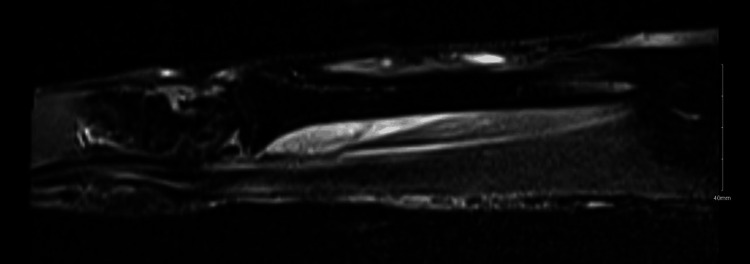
Distal FPL edema at the level of the distal radial diaphysis is evident on the MRI FPL, flexor pollicis longus
